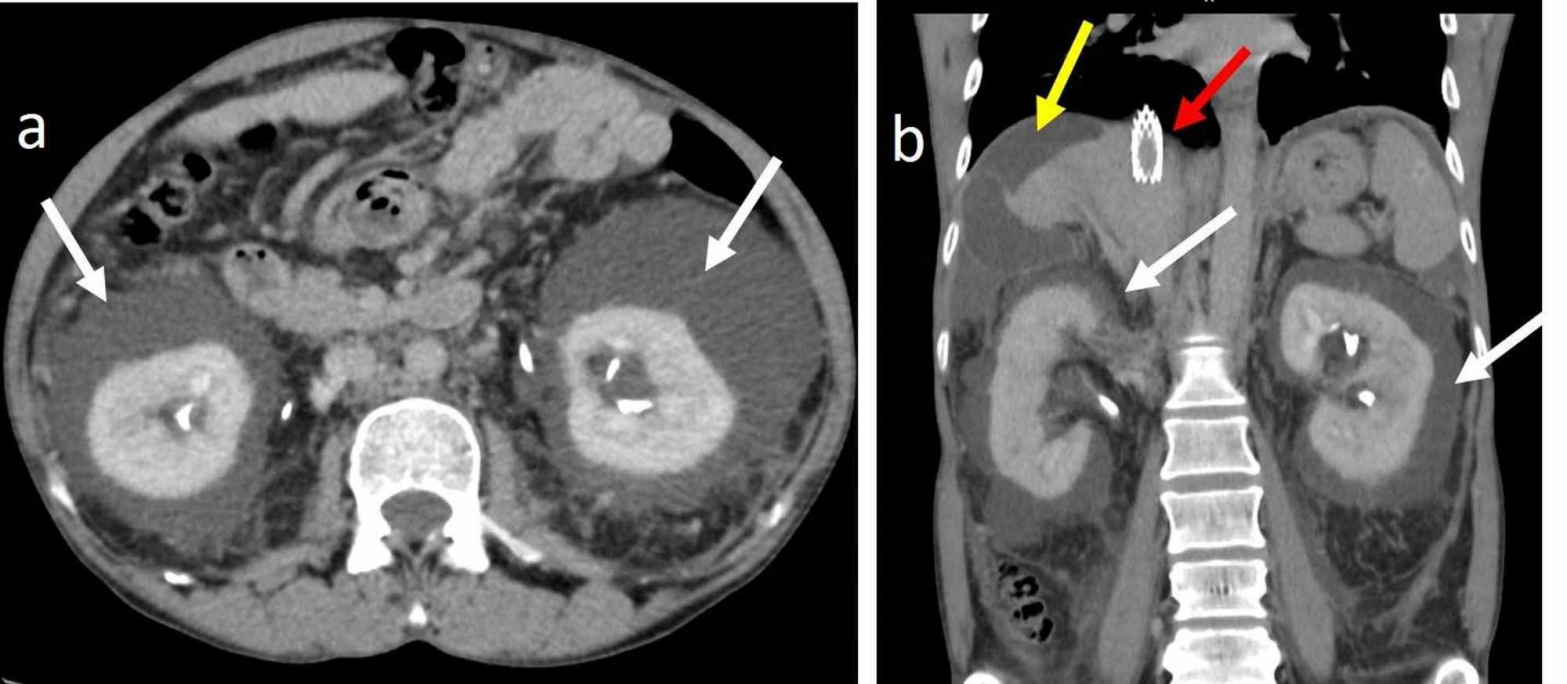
43-year-old male with bilateral perinephric renal lymphangiectasia (same patient as in Figure 4) Axial (a) and coronal (b) contrast-enhanced CT of the abdomen in the delayed phase demonstrates bilateral perinephric fluid collections (white arrows). The coronal image also demonstrates ascites (yellow arrow) and IVC stent (red arrow) CT: computed tomography; IVC: inferior vena cava
